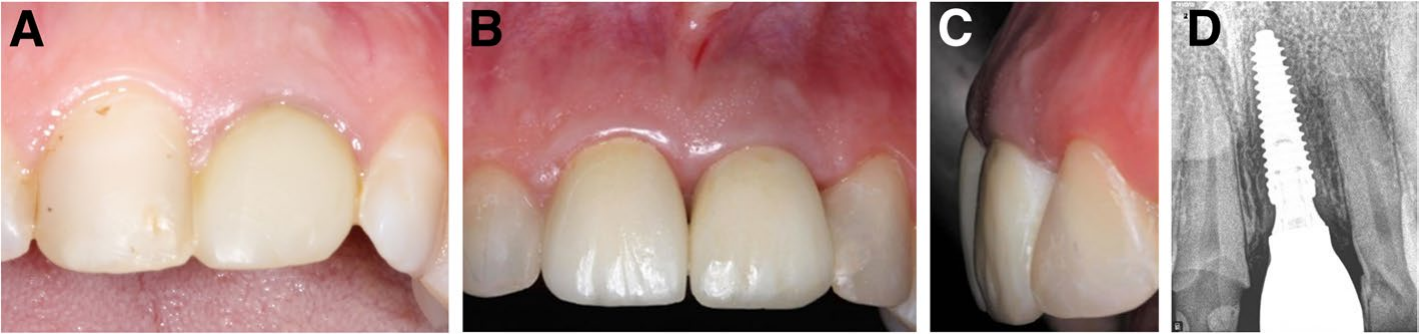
A-D: Photos and a radiograph of case #4 replacing the maxillary left central incisor. a Preoperative frontal view; b frontal view of the definitive crown at 12 months postloading; c lateral view of the definitive crown and peri-implant soft tissue at 12 months postloading; and (d) periapical radiograph at 12 months postloading
